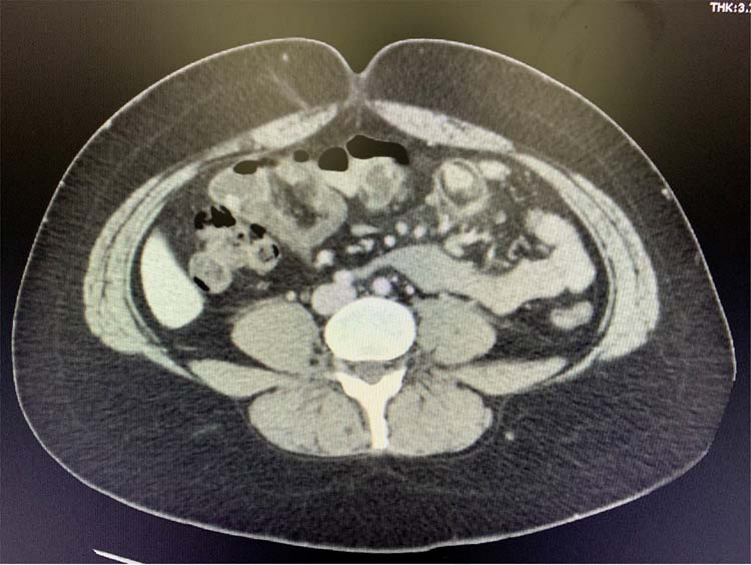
CT consistent with small bowel intussusception and inflammatory changes at the mesentery.
